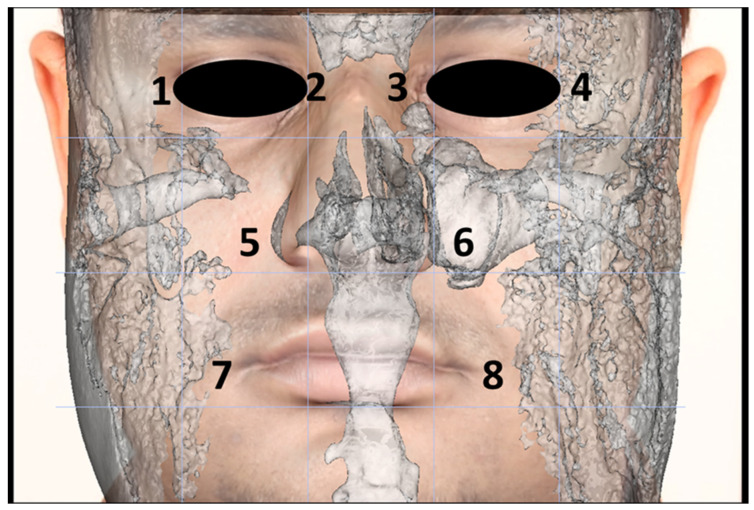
The eight CBCT wrapping points: (1) right lateral canthus, (2) right medial canthus, (3) left medial canthus, (4) left lateral canthus, (5) right nasolabial creases, (6) left nasolabial creases, (7) right oral commissure, and (8) left oral commissure.
